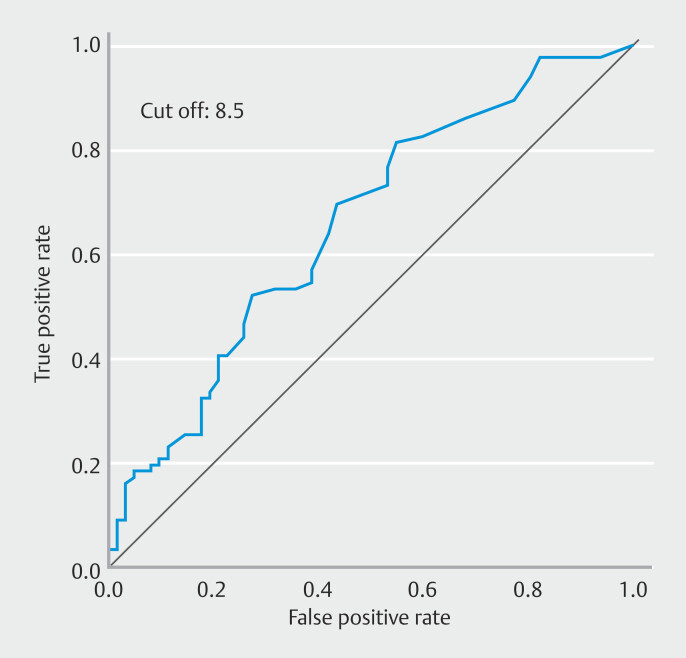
Analysis of average time from date of CT to date of SBCE. Logistic regression analysis determined the cutoff value to be 8.5 days (AUC 0.657).
